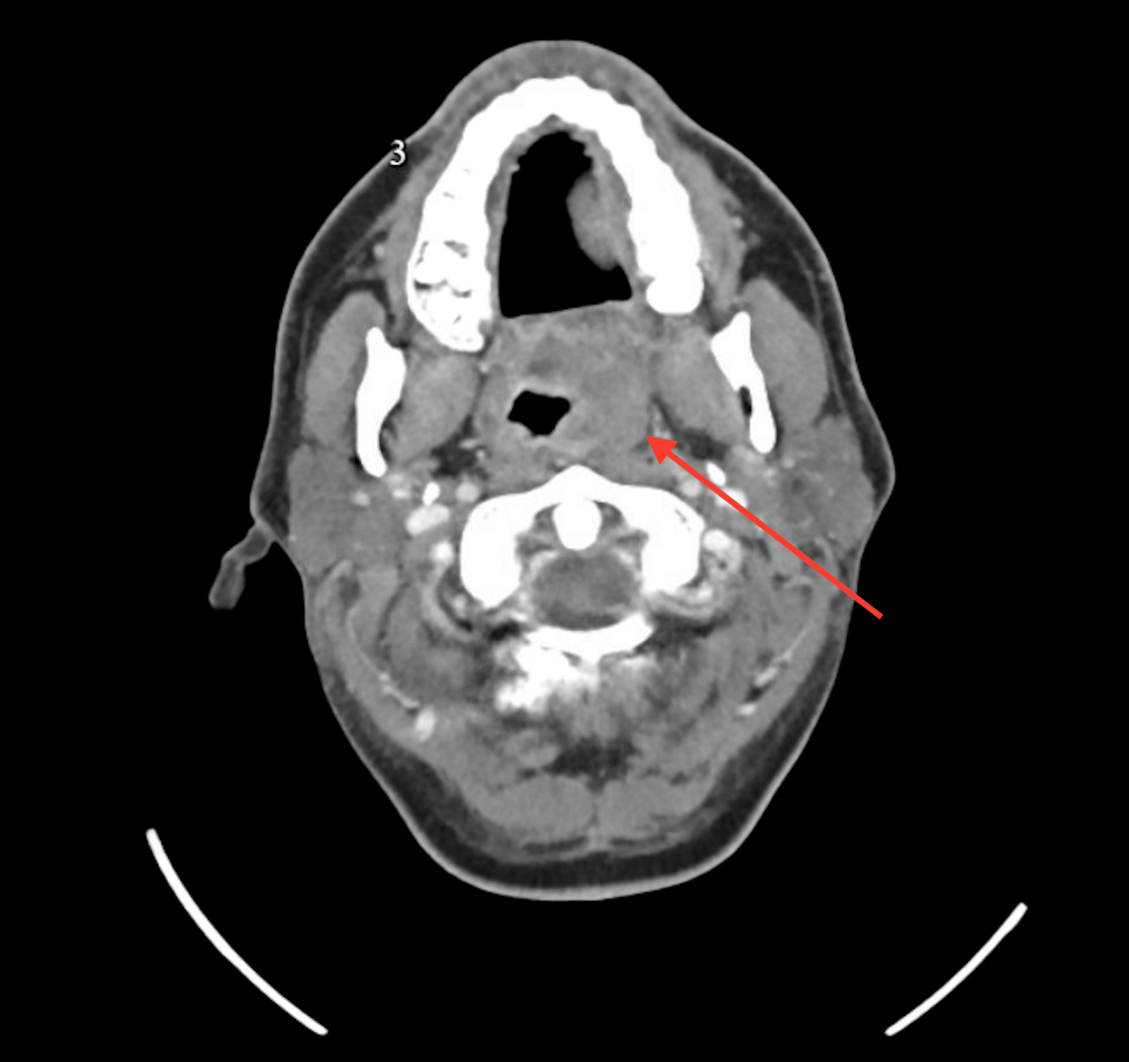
Contrast-enhanced axial CT of the neck demonstrating hypertrophy of lymphoid tissue and a parapharyngeal abscess on the left measuring 2.0 x 1.8 cm indicated by the arrow.
